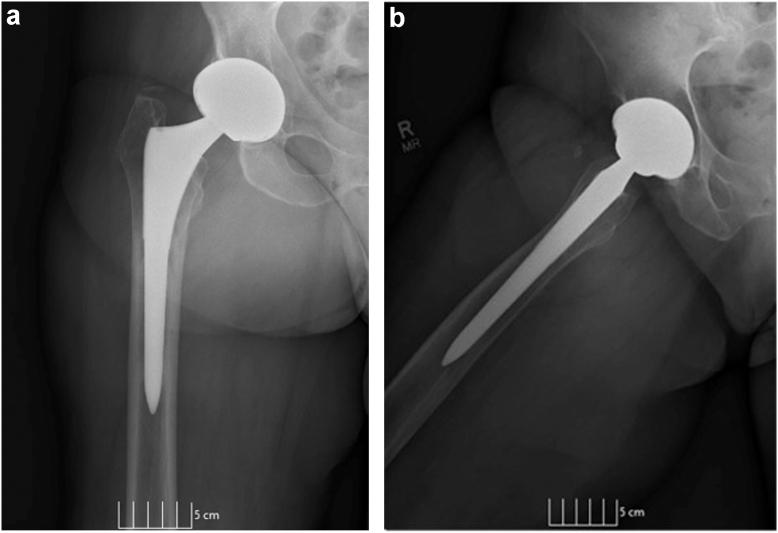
(a) Anterior-posterior (AP) and (b) frog lateral radiographs of right hip showing metal-on-metal total hip replacement without obvious evidence of peri-implant osteolysis or other acute changes. Soft tissue swelling was noted in the inguinal region.
